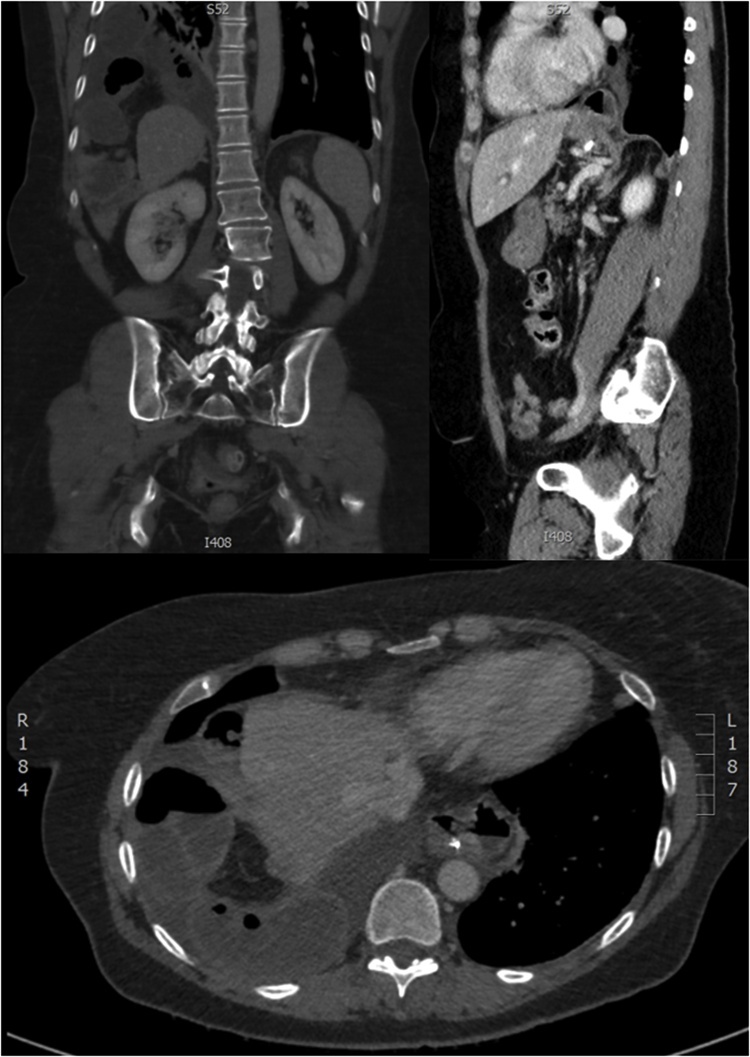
CT images showing defect.
